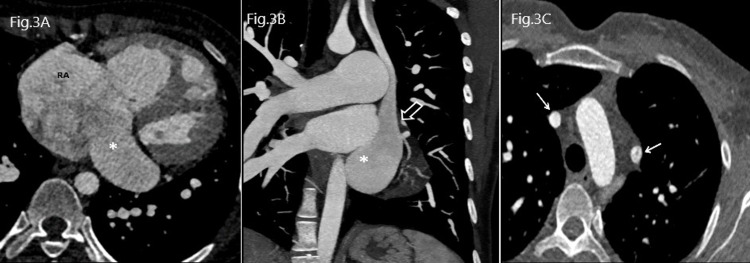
Section A: Contrast-enhanced CCT in axial view showing dilated coronary sinus (asterisk), Section B: Coronal maximum intensity projection (MIP) images view with dilated coronary sinus (asterisk) and left-sided superior vena cava draining into it (open white arrow), Section C: Contrast-enhanced CCT in axial view showing duplicated superior vena cava (white arrows) CCT: Cardiac Computed Tomography
